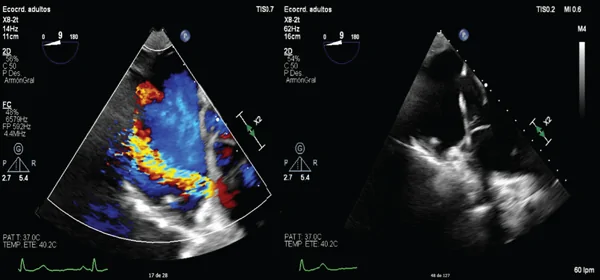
Mid‐esophageal transesophageal echocardiography view at 9° focused on the right heart chambers. A linear hyperrefringent structure is seen traversing the right atrium and perforating the anterior leaflet of the tricuspid valve, resulting in severe tricuspid regurgitation. Color Doppler imaging shows an eccentric regurgitant jet directed toward the lateral right atrial wall (Coanda effect).
